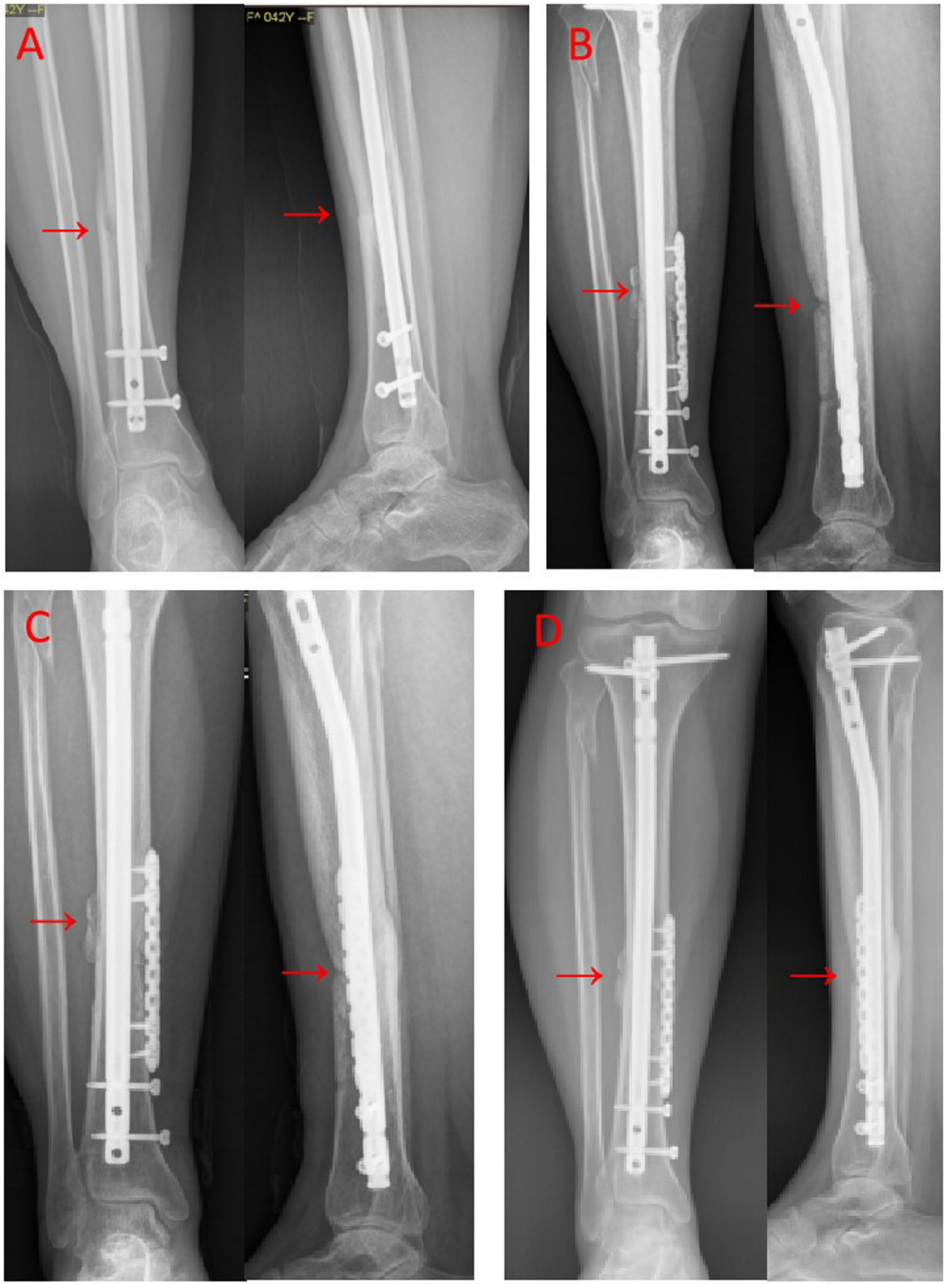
(A) After 9 months following closed reduction and intramedullary nail fixation of the fracture in the middle of the right tibia, anterior-lateral radiographs revealed nonunion. (B) After 3 months of PRP, augmentation plating and autogenous iliac bone implantation, the anterior-lateral x-ray images demonstrated favorable alignment with a slight increase in callus formation and a hazy appearance. (C) 6 months after revision, anterior-lateral radiographs exhibited enhanced callus formation and increased callus on both sides of the fracture. (D) 9 months after revision, anterior-lateral x-rays revealed indistinct fracture lines and fully healed fracture ends. The location of the fracture line is clearly indicated by a red arrow.
